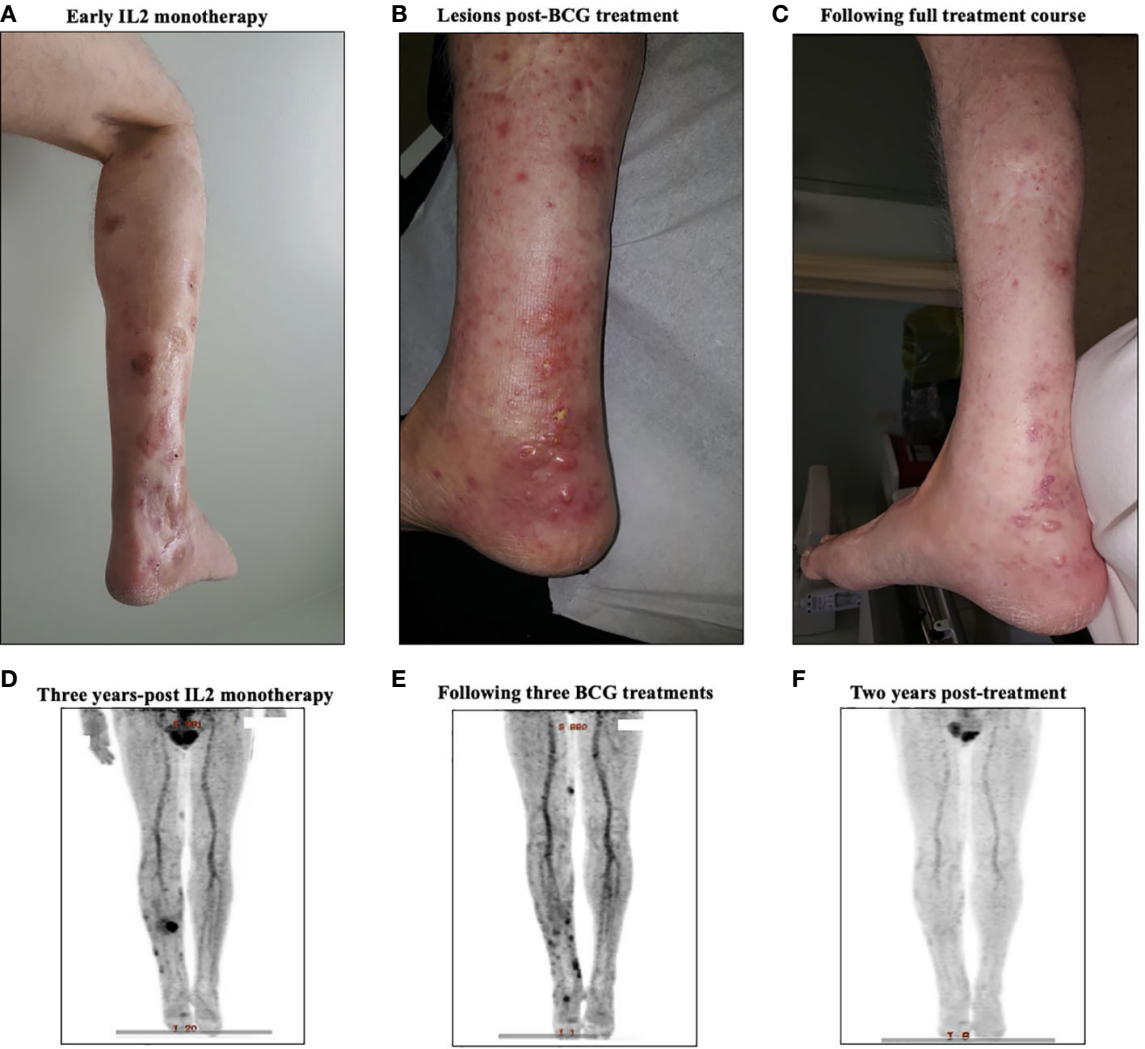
A representative case from a complete responder to intralesional IL-2 and BCG therapy. (A) Representative images of the treated lesion throughout IL-2 monotherapy treatment course. (B) PET-CT scan three years into IL-2 monotherapy showing new uptake among cutaneous lesions, signaling disease progression. (C) Representative image of the treated lesion following IL-2 and BCG treatment. (D) PET-CT scan after three BCG treatments showing regression of main lesions radiographically with new FDG uptake in distal lesions. (E) Representative image of the area after treatment, showing clinical resolution of BCG ulcers and in-transit metastasis. (F) Surveillance PET-CT two years post-treatment, showing no evidence of disease.
